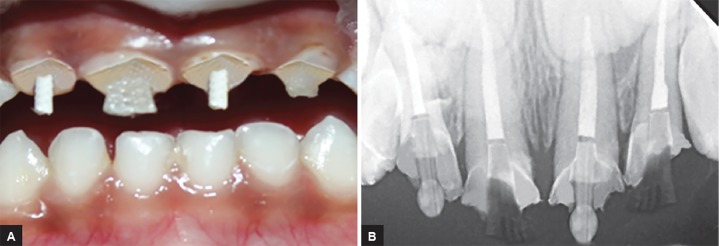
(A) Post placement clinically; and (B) radiographically
